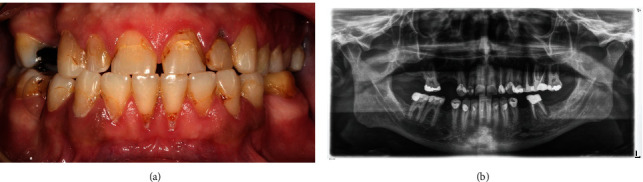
(a) Intraoral frontal view of the patient teeth preoperatively. (b) Panoramic X-ray view of the patient teeth preoperatively.
